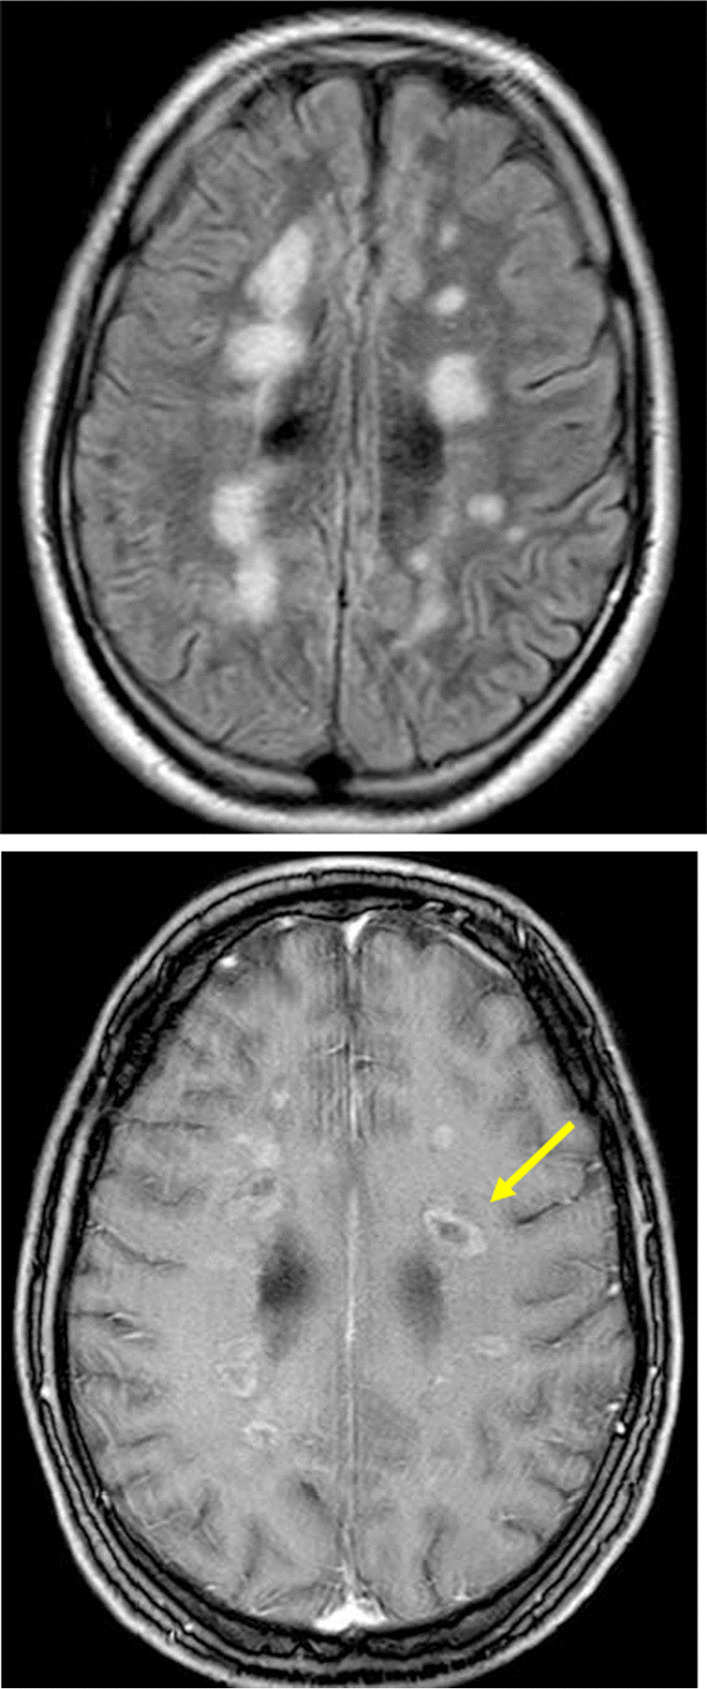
Follow-up MRI 2 weeks after initial admission shows an increase in size of the white matter lesions on the FLAIR sequence, representing further swelling of the brain tissue. Most lesions showed a ring shaped contrast enhancement (Arrow)
